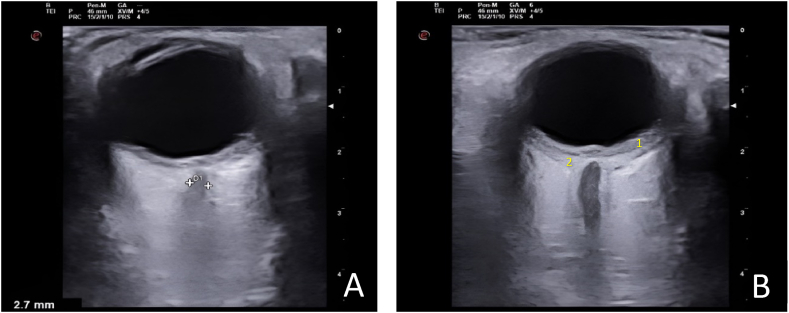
Orbital ultrasound at the time of admission*. It shows a diffuse posterior choroidal thickening [left eye (B) > right eye (A)] together with an accumulation of subretinal fluid. These ultrasound findings suggested the existence of bilateral chorioretinitis: o *1.Diffuse posterior choroidal thickening* o *2.An accumulation of subretinal fluid*.
